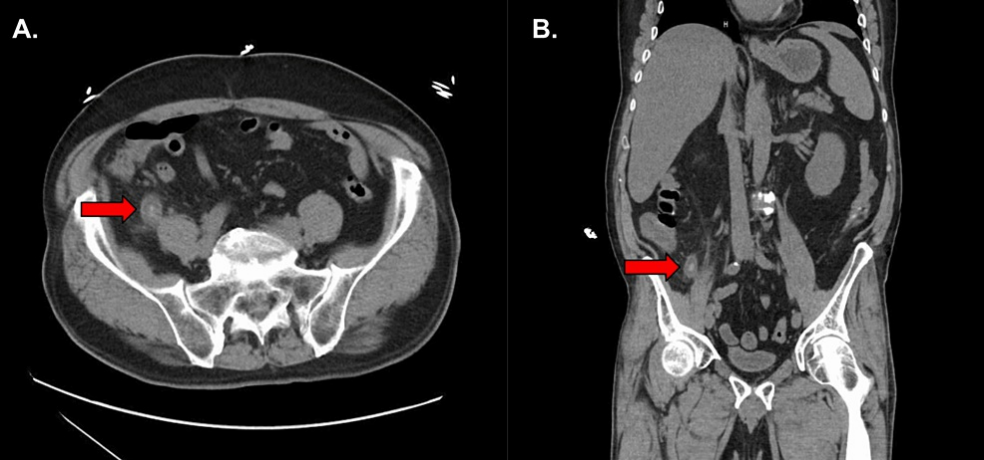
Preoperative CT revealing suspected appendicitis. Axial (A) and coronal (B) CT scan of abdomen and pelvis demonstrating periappendiceal inflammation and the classic target sign of appendicitis (red arrow). CT, computed tomography
